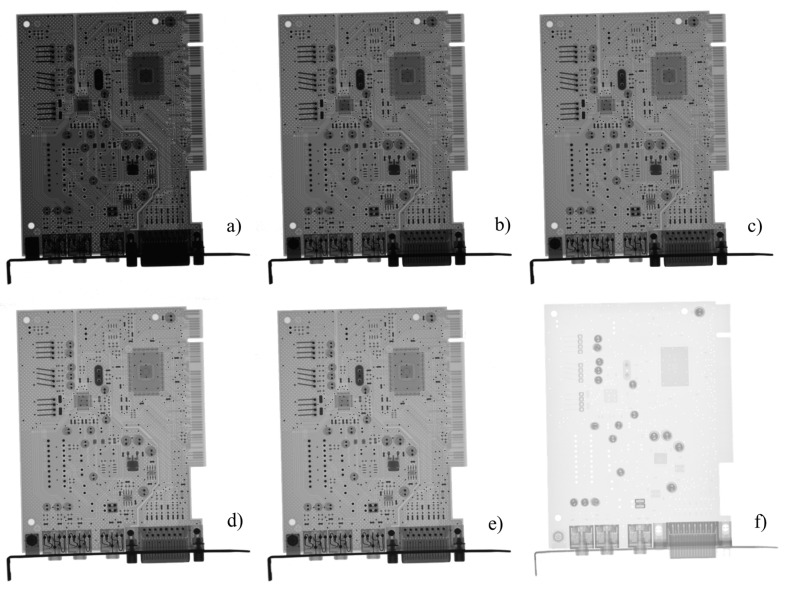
X-ray radiograms of a Creative Sound Blaster PCI 128 type sound card taken with X-ray tube voltages of (a) 50, (b) 75, (c) 100, (d) 150, (e) 200 kV, and the corresponding (f) thermal neutron image. Note that different structural features are visible with the different exposure conditions.
